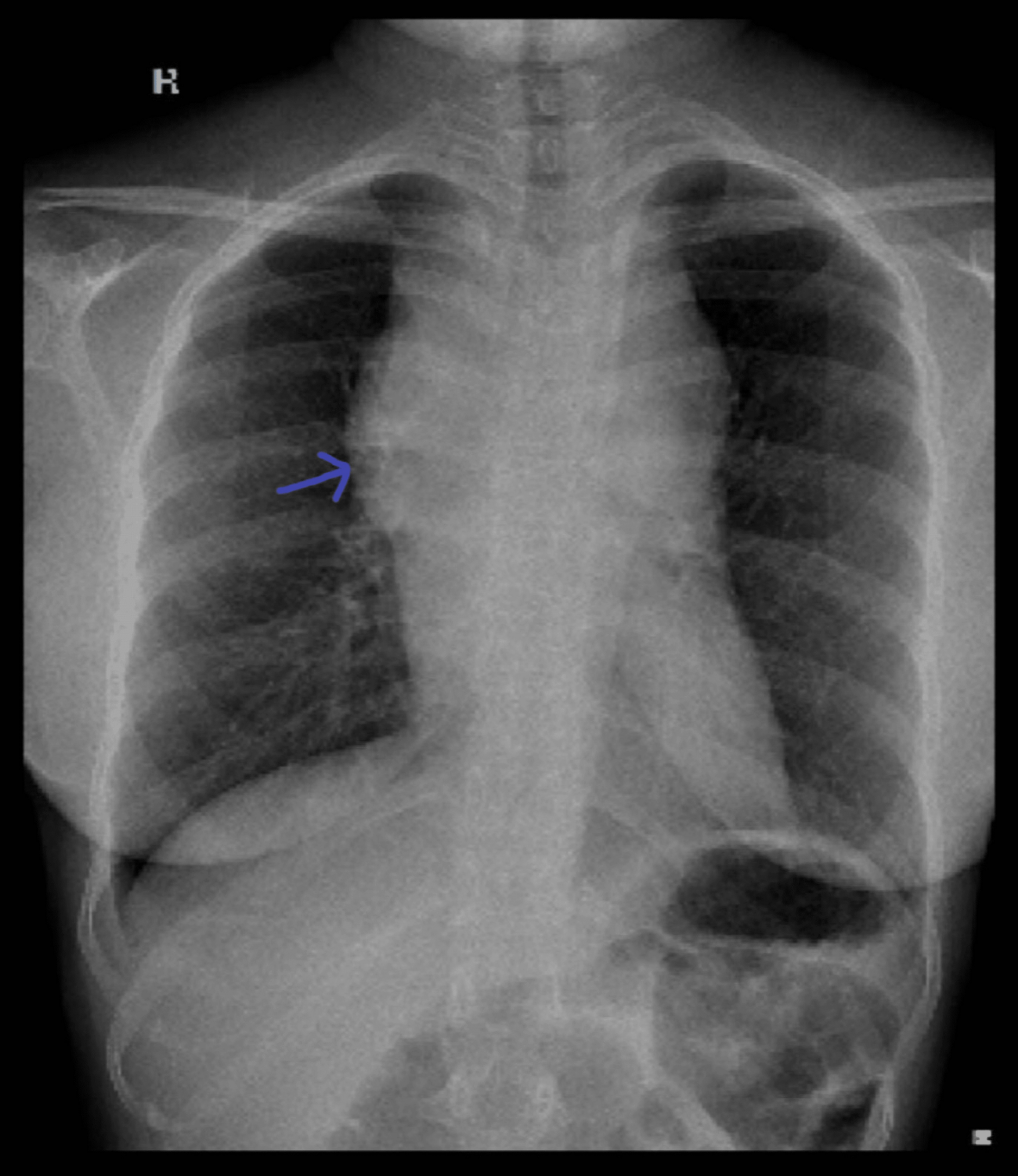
Chest X-ray posteroanterior view of chest X-ray showing features of a mediastinal mass, more prominent over the right side, widening of the superior mediastinum, and obscuration of the right cardiac border.
